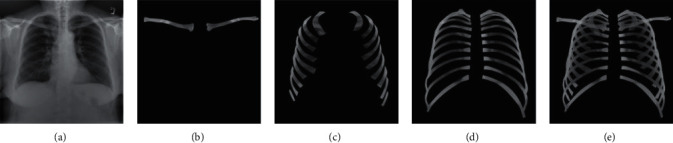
A raw chest X-ray and the corresponding parts for segmentation, including the clavicle, anterior ribs, posterior ribs, and all bones (the complete set of ribs and clavicles). (a) Chest radiograph. (b) Image of the clavicles. (c) Image of the anterior ribs. (d) Image of the posterior ribs. (e) Image of all bones.
